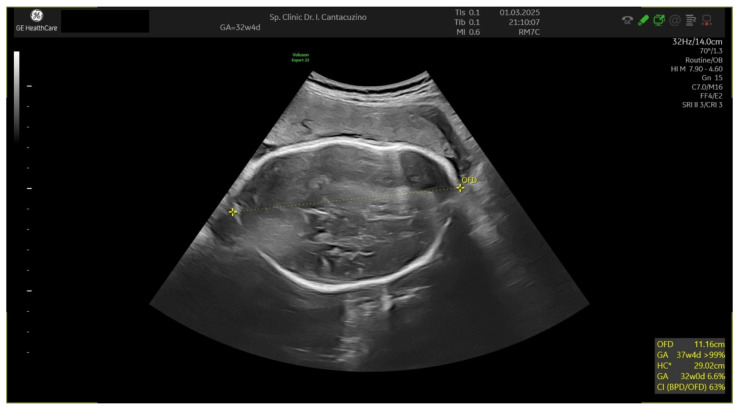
Ultrasound aspect of fetal cranium.
